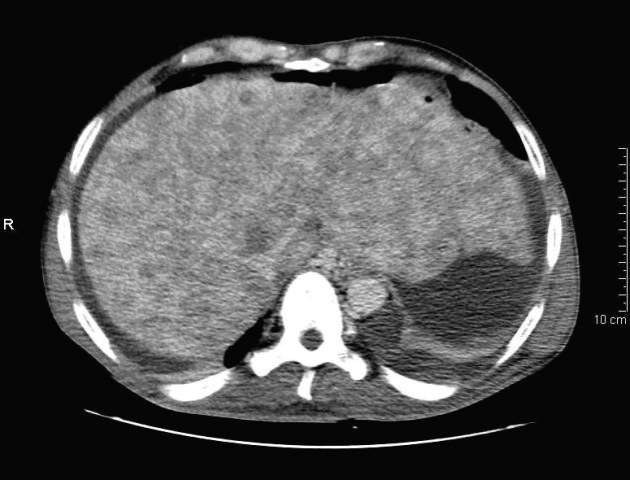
First abdominal computed tomography of our patient showing ascites and diffuse heterogeneous liver nodules, filled with contrast-like agent.
